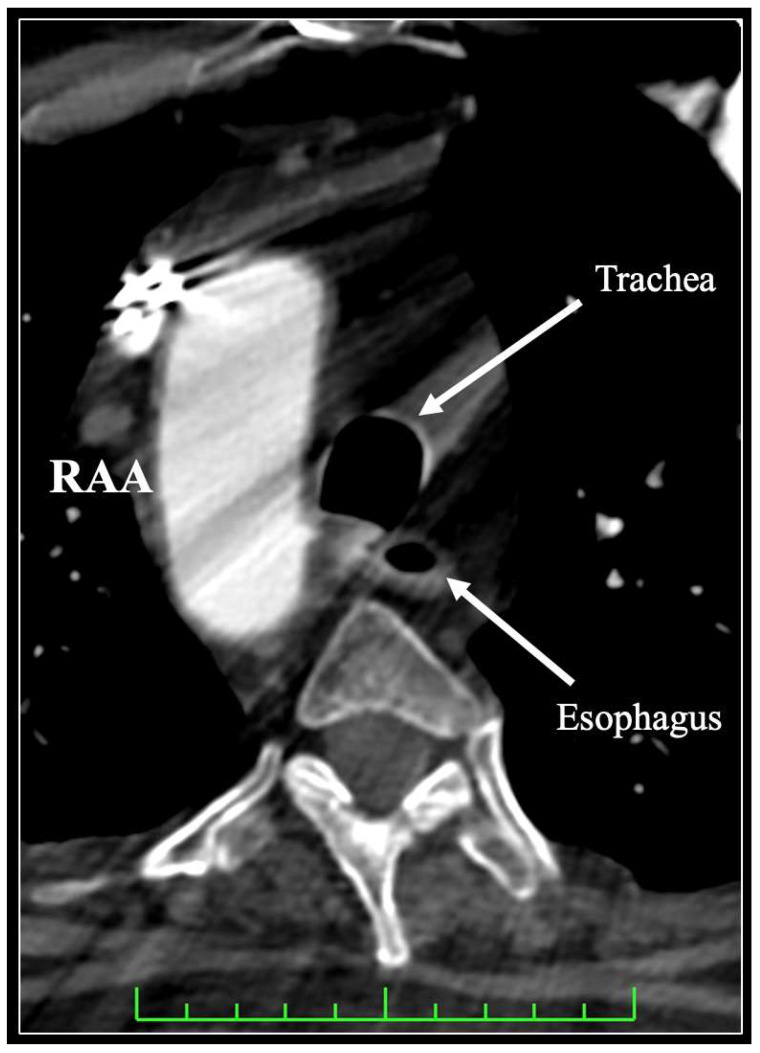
Computed tomography angiography of right-sided aortic arch (RAA) demonstrated in axial reconstruction.
